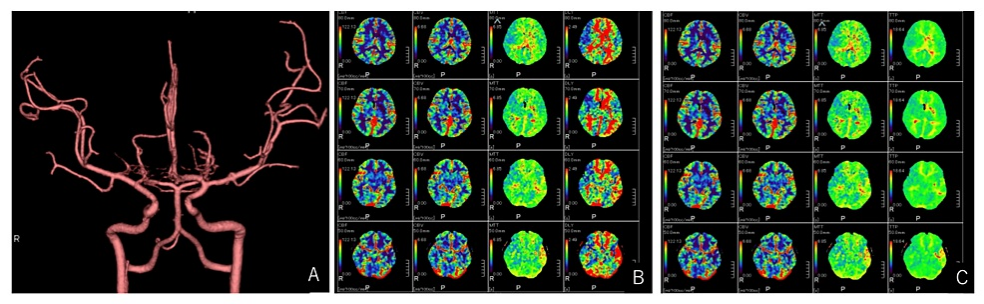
Computed tomography (CT) angiography and perfusion imaging after admission. CT angiography on the day after admission displayed no progression of arterial dissection (A). CT perfusion on the same day revealed decreased cerebral blood flow and cerebral blood volume at the infarct site. Still, cerebral blood flow in the peripheral portion of the middle cerebral artery was preserved (B, C).
